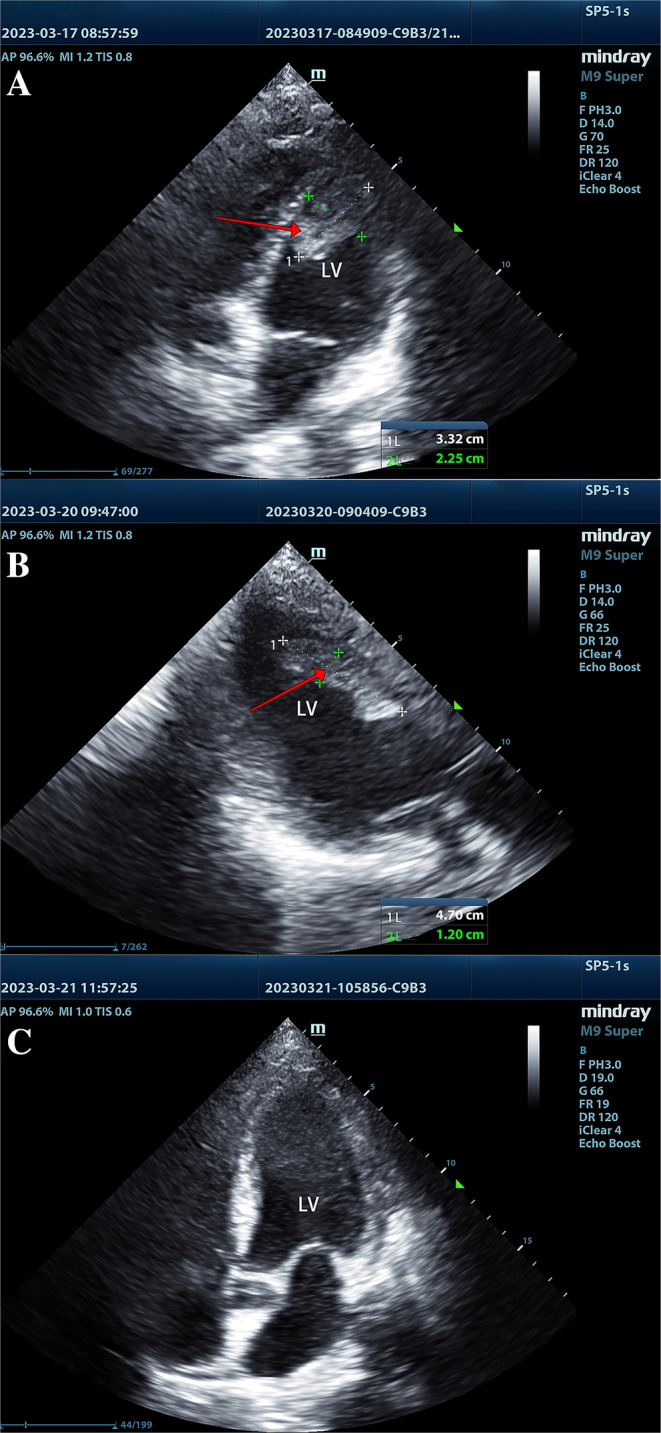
(A) Showing that on March 17, 2023, an echocardiogram revealed a left ventricular thrombus measuring 33 × 23 mm. (B) Showing that the maximum size of the thrombus (47 × 12 mm) was measured on March 20, 2023. (C) Showing that the left ventricular thrombus on March 21, 2023.
